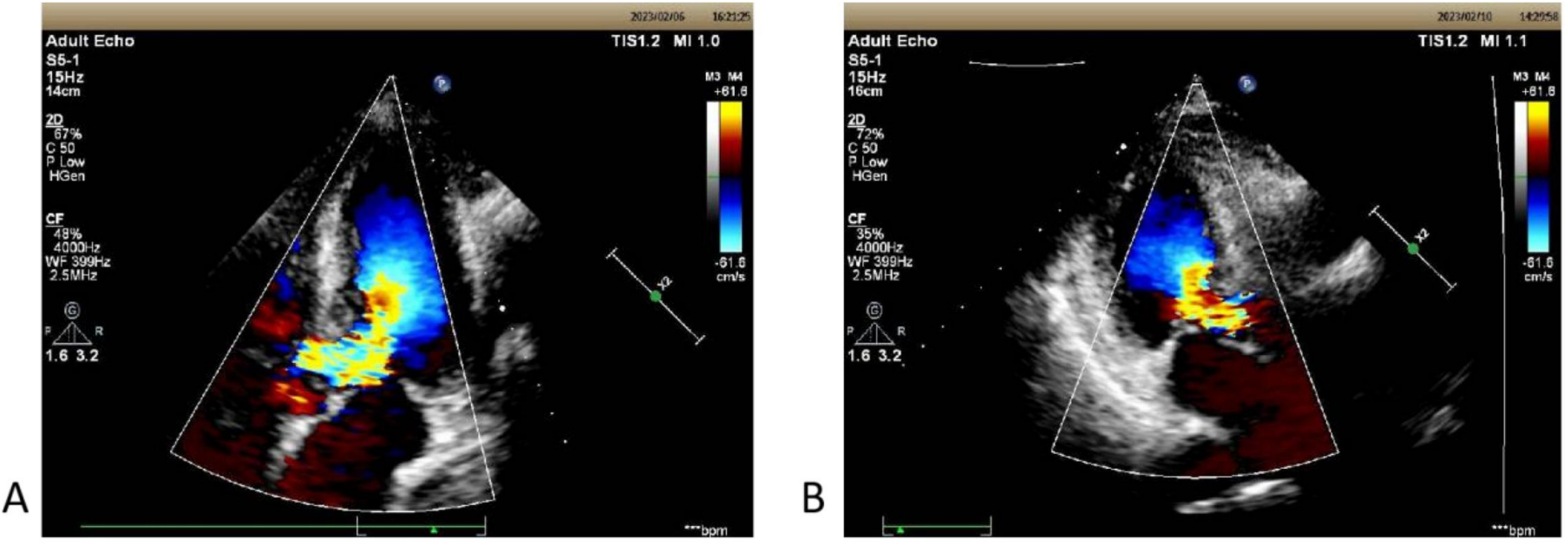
(A) The echocardiographic view of patient 1 before operation with septal hypertrophy measuring 1.60 cm. Severe left ventricular outflow tract obstruction with increased blood flow velocity in the left ventricular outflow tract at rest, maximum flow velocity of 528 cm/s, maximum pressure gradient of 111 mmHg, and mean pressure gradient of 51 mmHg. (B) On the third postoperative day, the second echocardiography showed: The left ventricular outflow tract velocity with a maximum flow velocity of 244 cm/s and a maximum pressure gradient of 24 mmHg.
